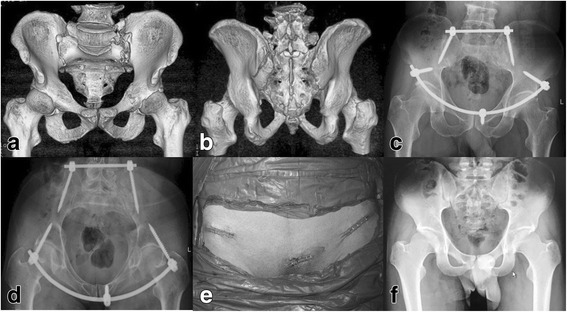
A 57-year-old male patient with anterior and posterior pelvic ring fracture because of a crushing injury. a, b Preoperative 3-D CT image showing the anterior pelvic ring fracture. c, d Postoperative X-ray film showing the satisfactory reduction with modified pedicle screw-rod fixation (modified PSRF). e The postoperative incision. f X-ray film showing the healed fracture at postoperative 8 months
